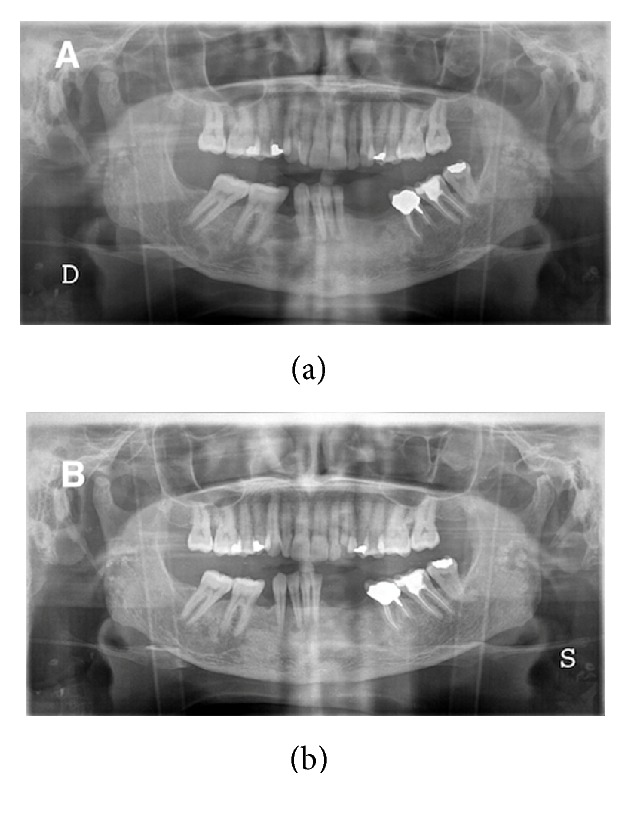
Radiographic appearance after four months and two years. (a) Signs of bone healing after four months after surgical excision of the parathyroid adenoma. (b) Complete recovery of the bony tissue two years after the operation.
